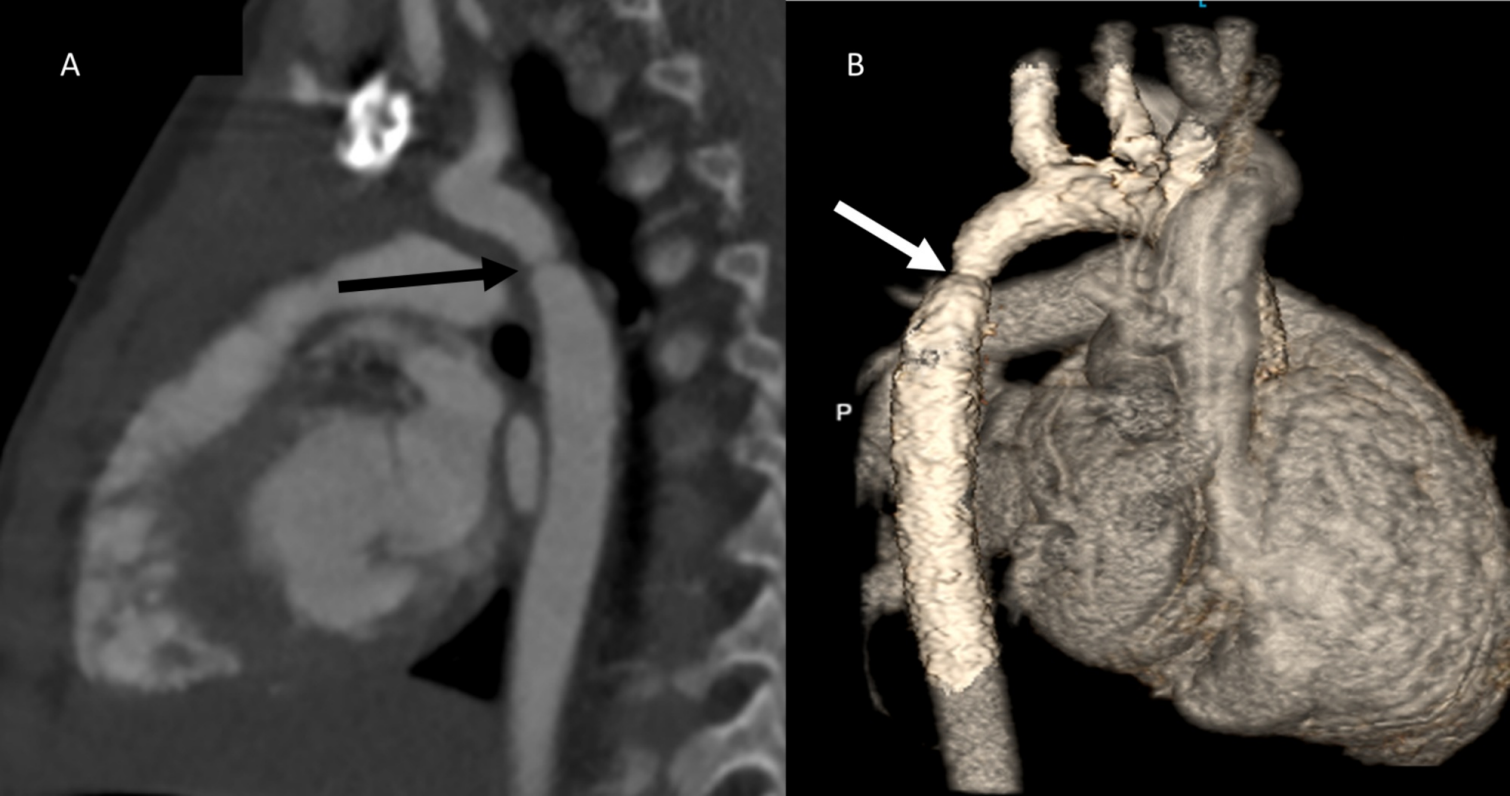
Computed tomography scan imaging of coarctation or aorta. (A) Two-dimensional sagittal reconstruction showing site of discrete narrowing at the level of aortic isthmus (arrow). (B) Three-dimensional reconstruction of computed tomographic angiography showing site of discrete narrowing in the same patient when viewed from posterior aspect (arrow).
